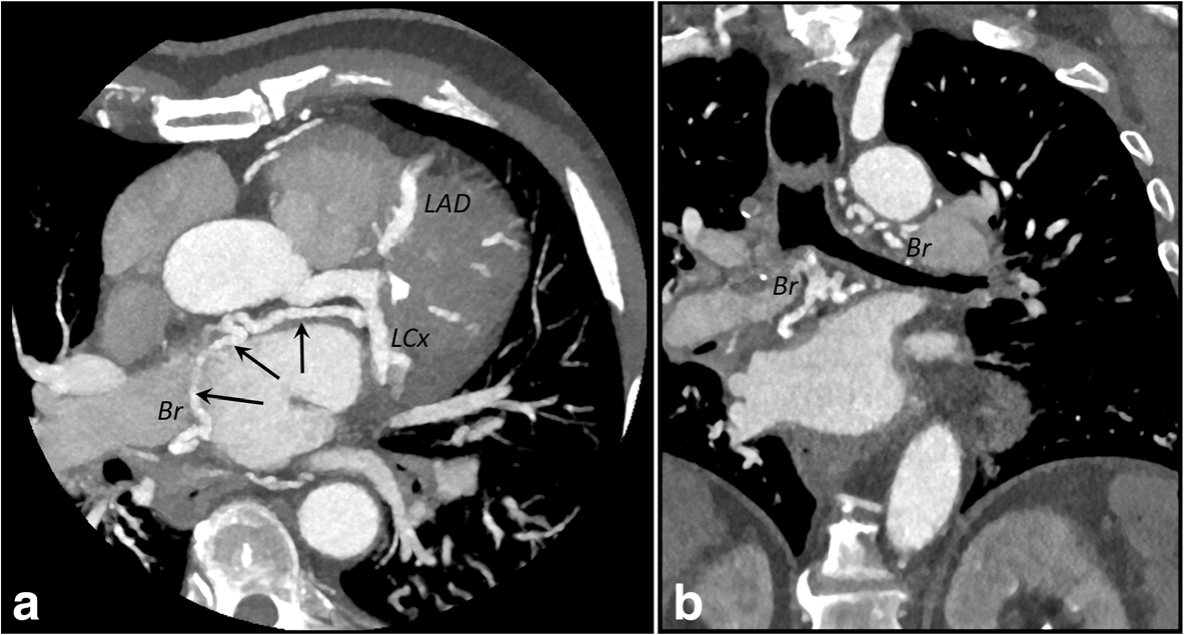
a and b Axial (a) and coronal (b) maximum-intensity projection (10 mm) coronary computed tomographic angiographic images showing dilated bronchial arteries (Br) anastomosing to the left circumflex artery (LCx) (*arrows*). *LAD* Left coronary artery
